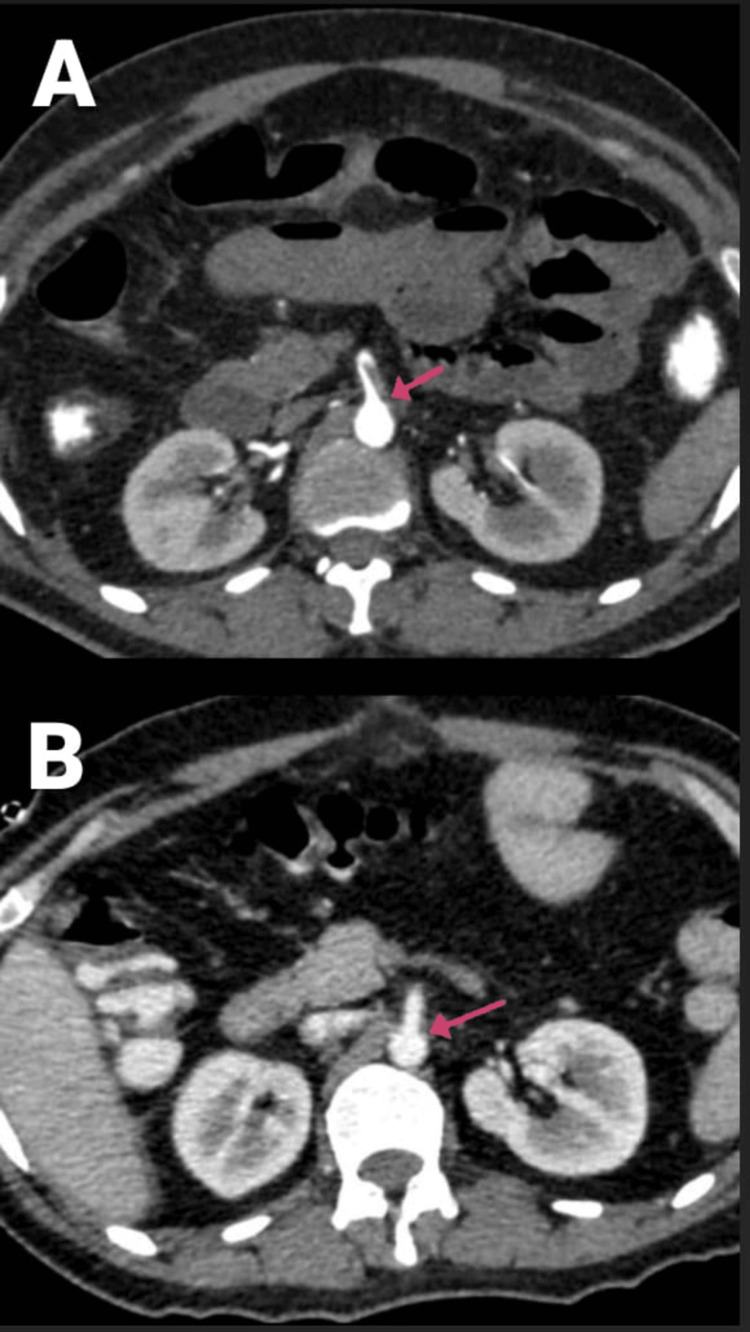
(A and B) CECT of the abdomen and pelvis with angiography of the patient (arrows showing a partial filling defect in the SMA along its posterior and left lateral wall). CECT, contrast-enhanced computed tomography; SMA, superior mesenteric artery
